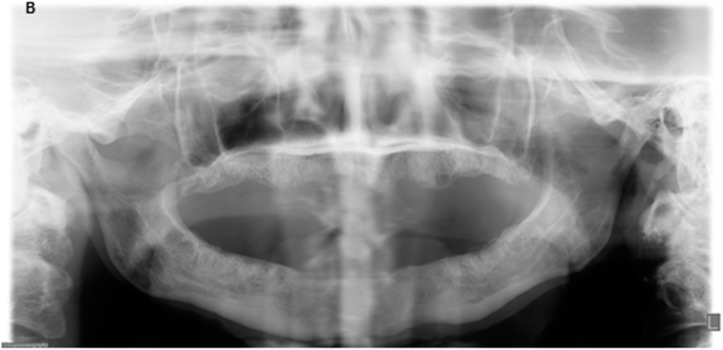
Radiologic findings before surgery and nine months after surgery. (A) Panoramic radiograph with extensive osteolysis, extending from region 46 to 34 up to the inferior border in the region of the symphyse; (B) Panoramic radiograph nine months post-operatively with healed, smooth edges of the mandibular body.
